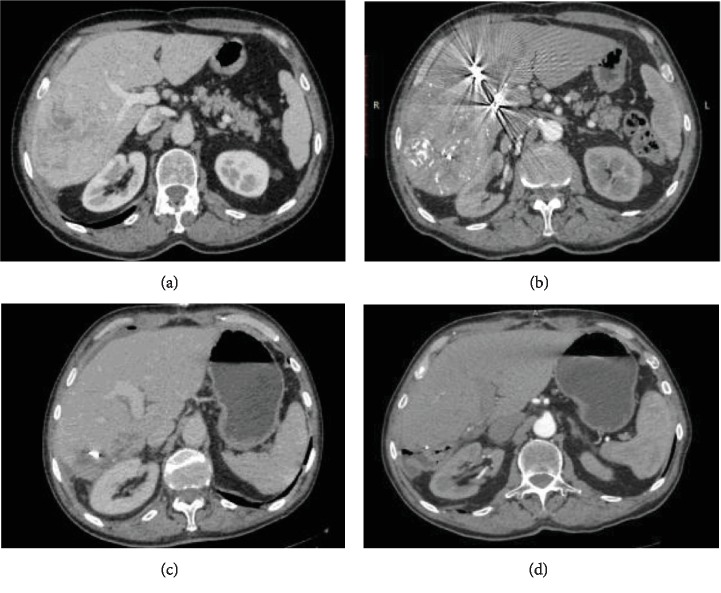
(a) CT scan before TACE and PVE. (b) One month after PVE and three months after TACE, anticipated hypertrophy of LLL is observed. On CT scan, one month (c) and three months (d) after liver resection, there is no evidence of HCC recurrence. Significant hypertrophy of the liver remnant is noted.
